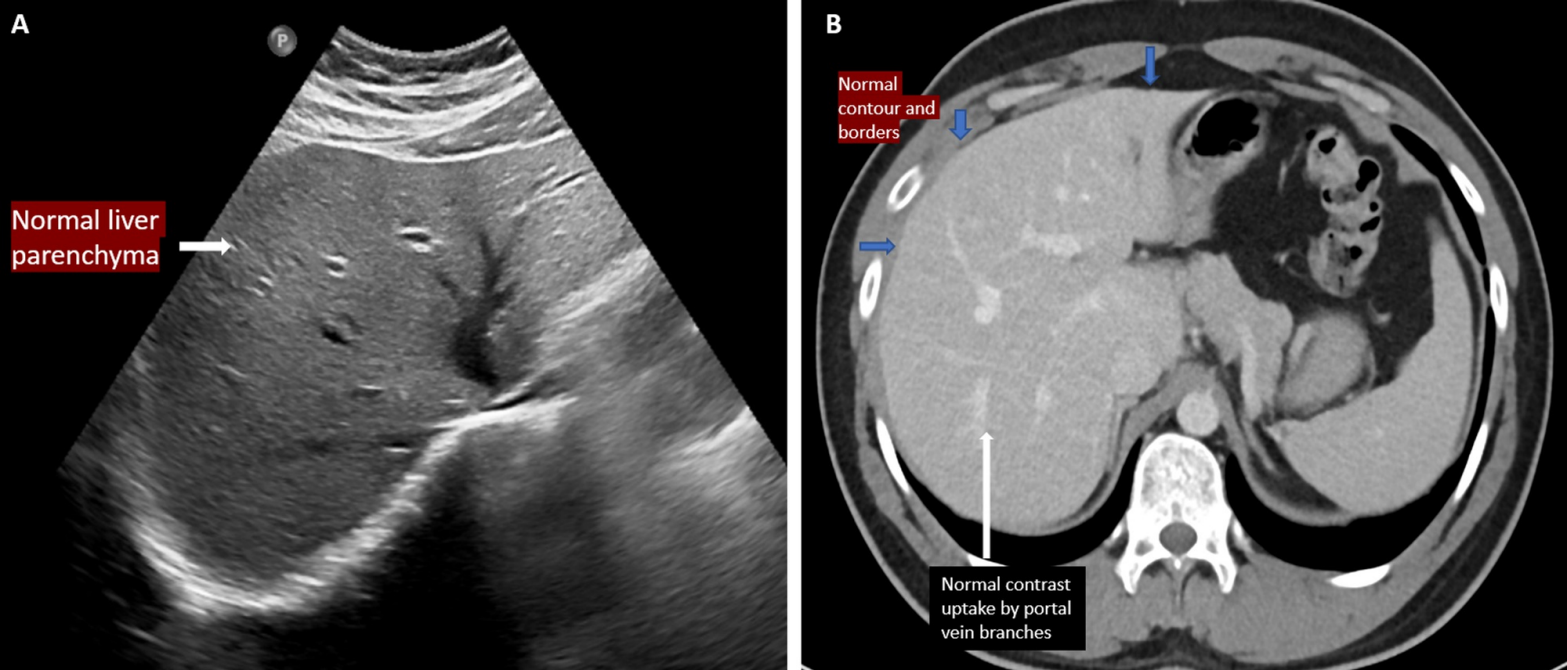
A selected image of a grey-scale ultrasound abdomen shows normal parenchymal echotexture, no focal lesion, or biliary dilatation (1A). A selected axial section of a contrast-enhanced CT scan of the abdomen demonstrates no focal lesion or collection in the liver or perihepatic fluid (1B).
